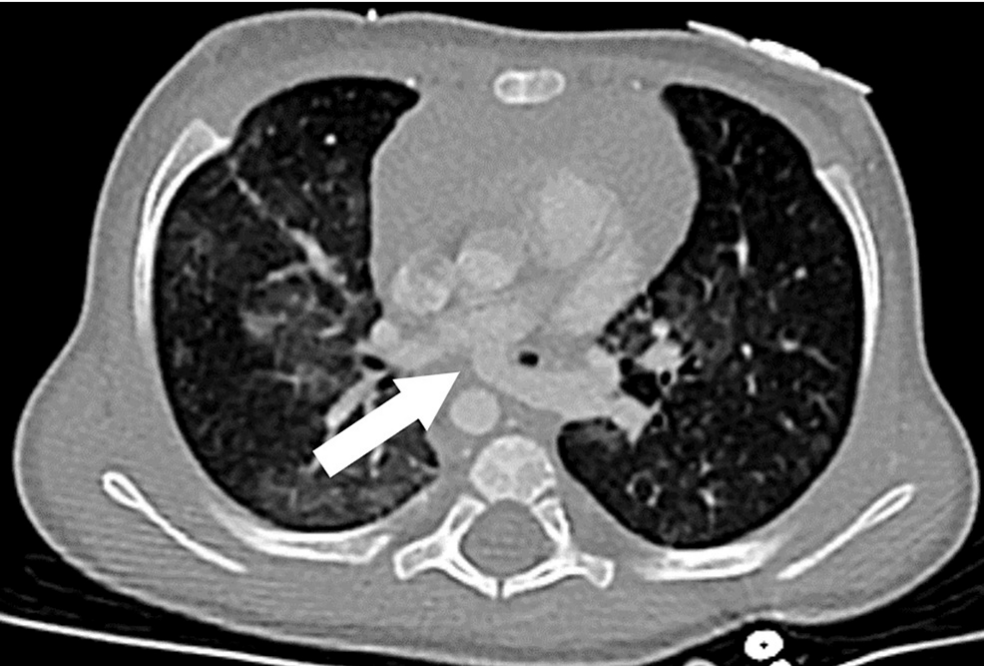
CT-imaging of LPA sling. The high-resolution chest CT imaging demonstrates the left pulmonary artery passing between the esophagus and trachea. LPA: left pulmonary artery.
